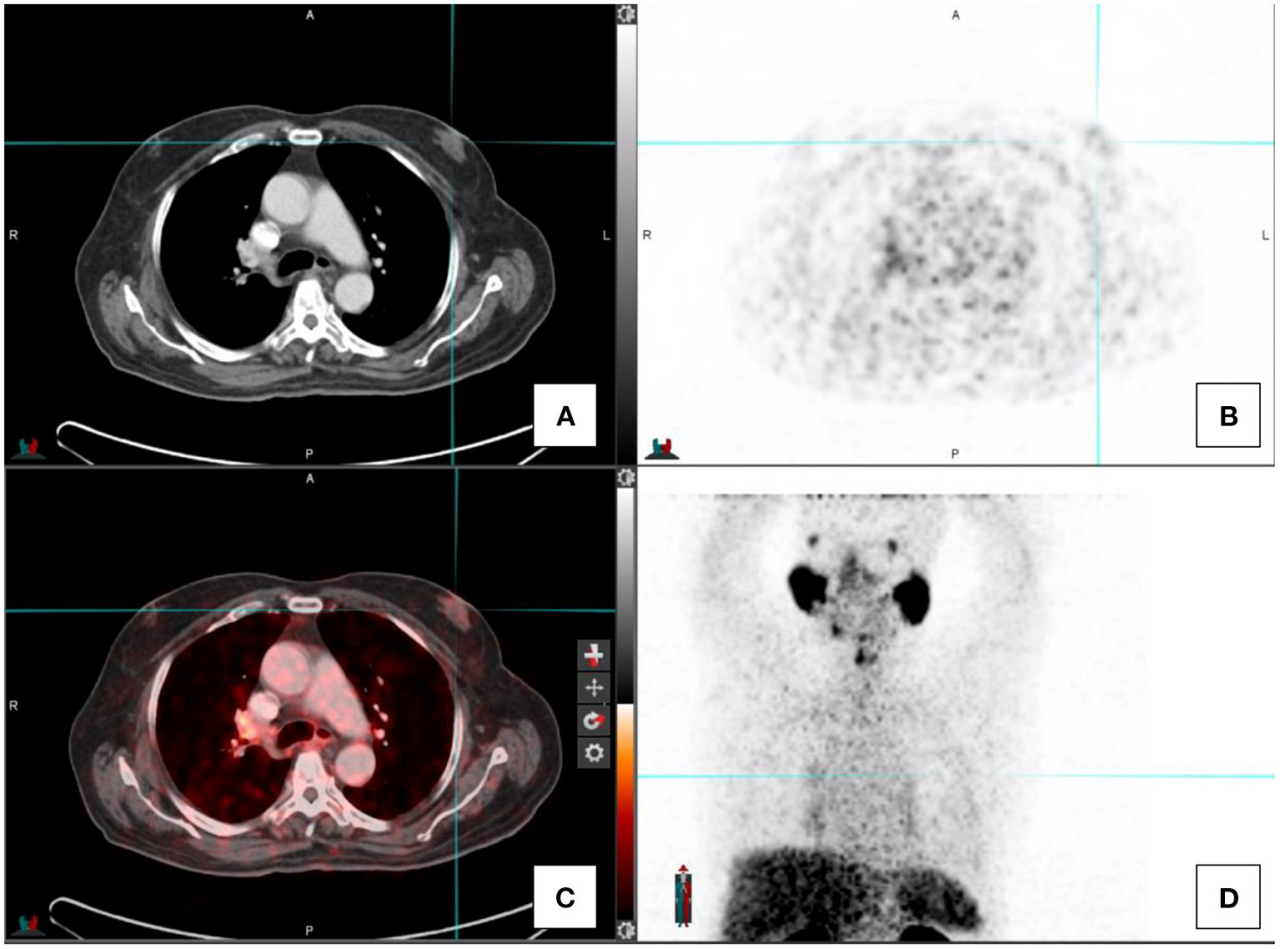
A patient with gynecomastia with associated low-grade Ga68-PSMA activity. CT-image (A), PET image (B), fused PET/CT image (C), and the maximal intensity projection image (D).
